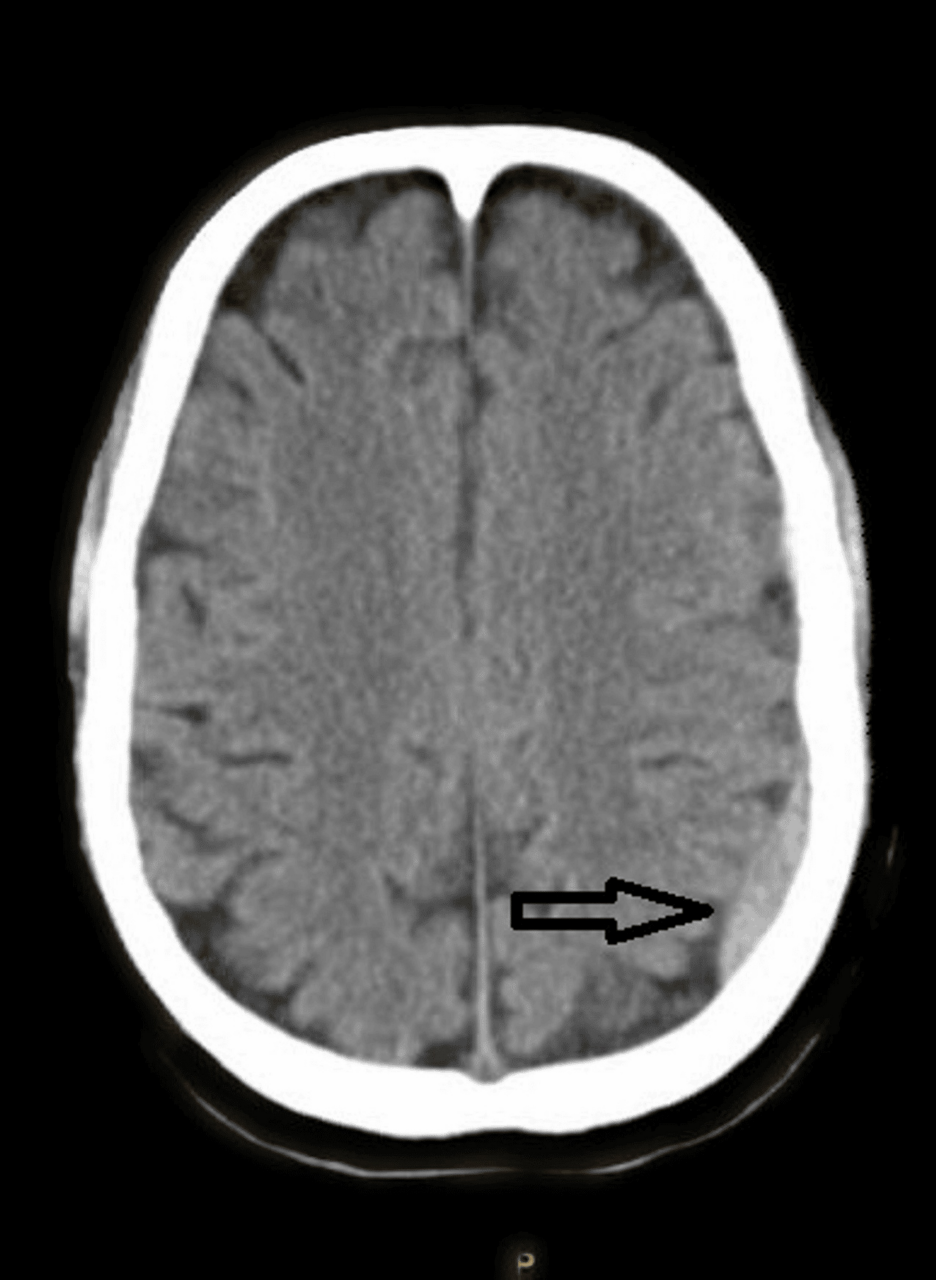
Non-contrast computed tomography of the brain showing a left parieto-occipital subdural hematoma
